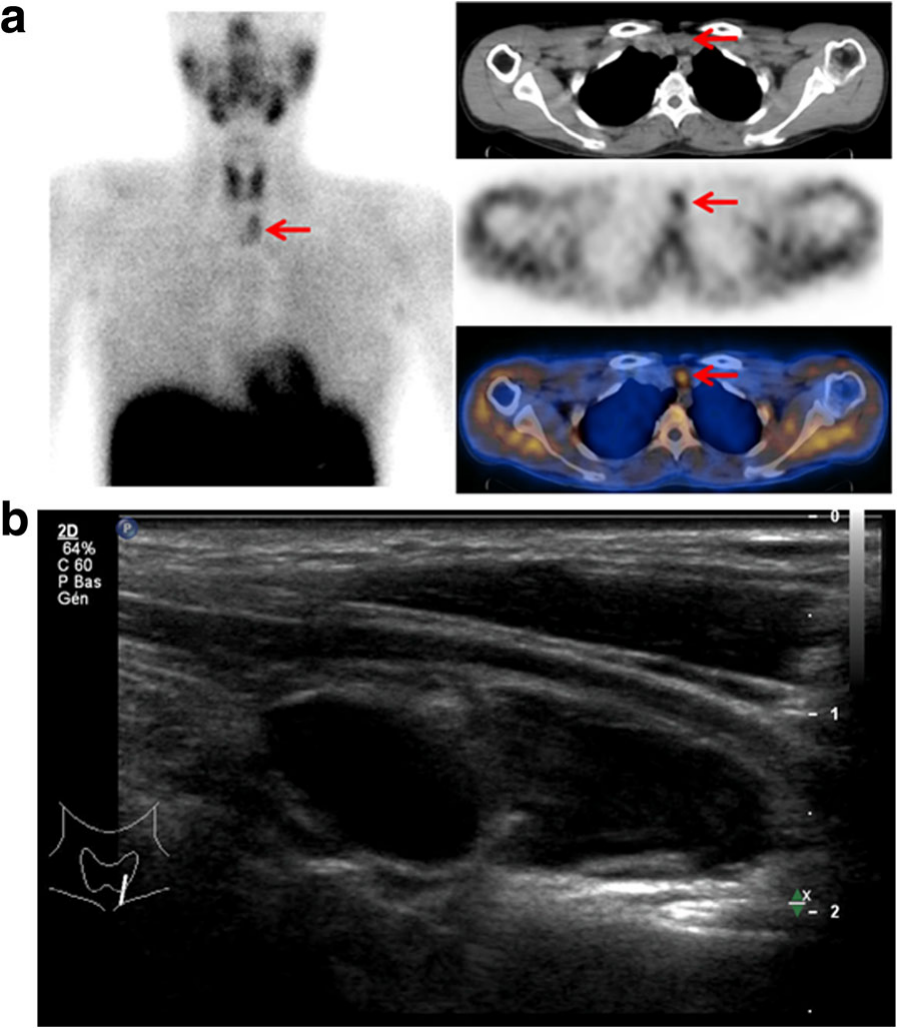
Patient 2. a Technetium 99 m sesta methoxyisobutylisonitrile scintigraphy (planar and single-photon emission computed tomography-computed tomography) showing a focal tracer retention localized under the left lower thyroid lobe just above the manubrium and anterior to the trachea (*red arrows*). b Cervical ultrasound with longitudinal view of a mixed solid and cystic nodule
